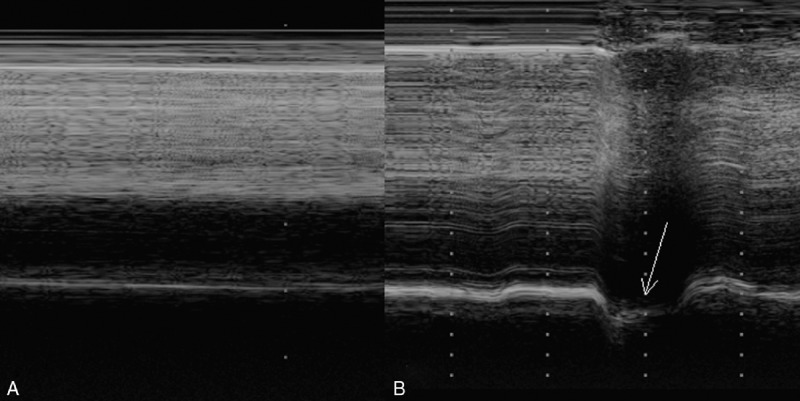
Right hemidiaphragmatic motion recorded in M-mode sonography: absence of movement during (a) quiet breathing and (b) paradoxical movement (arrow) during voluntary sniffing.
